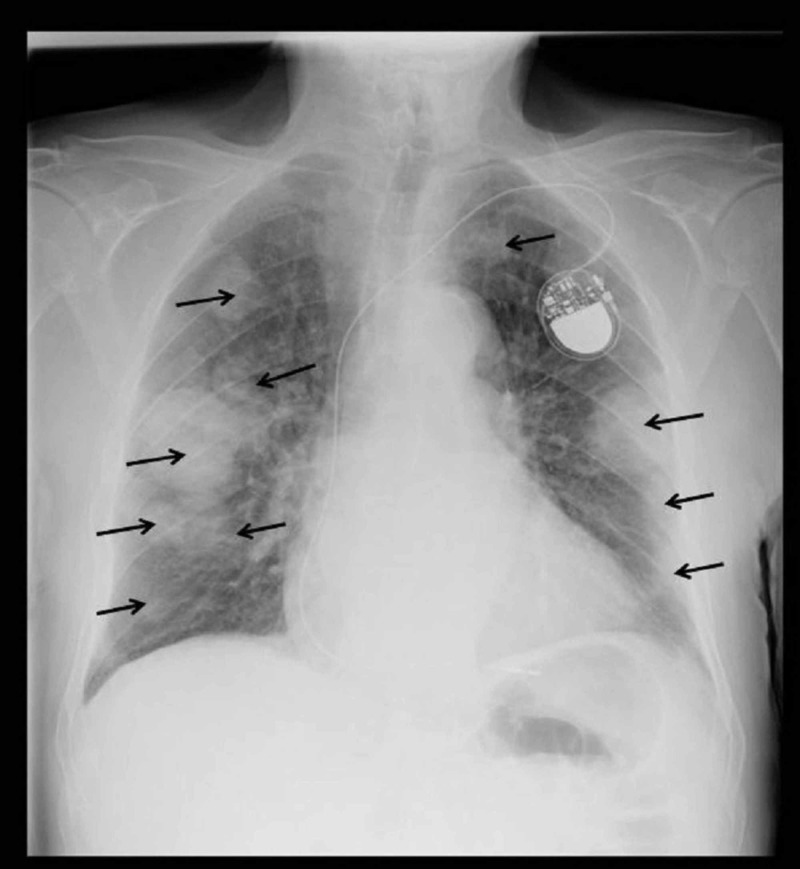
Chest X-ray Showing large bilateral lung field metastases (black arrows)
